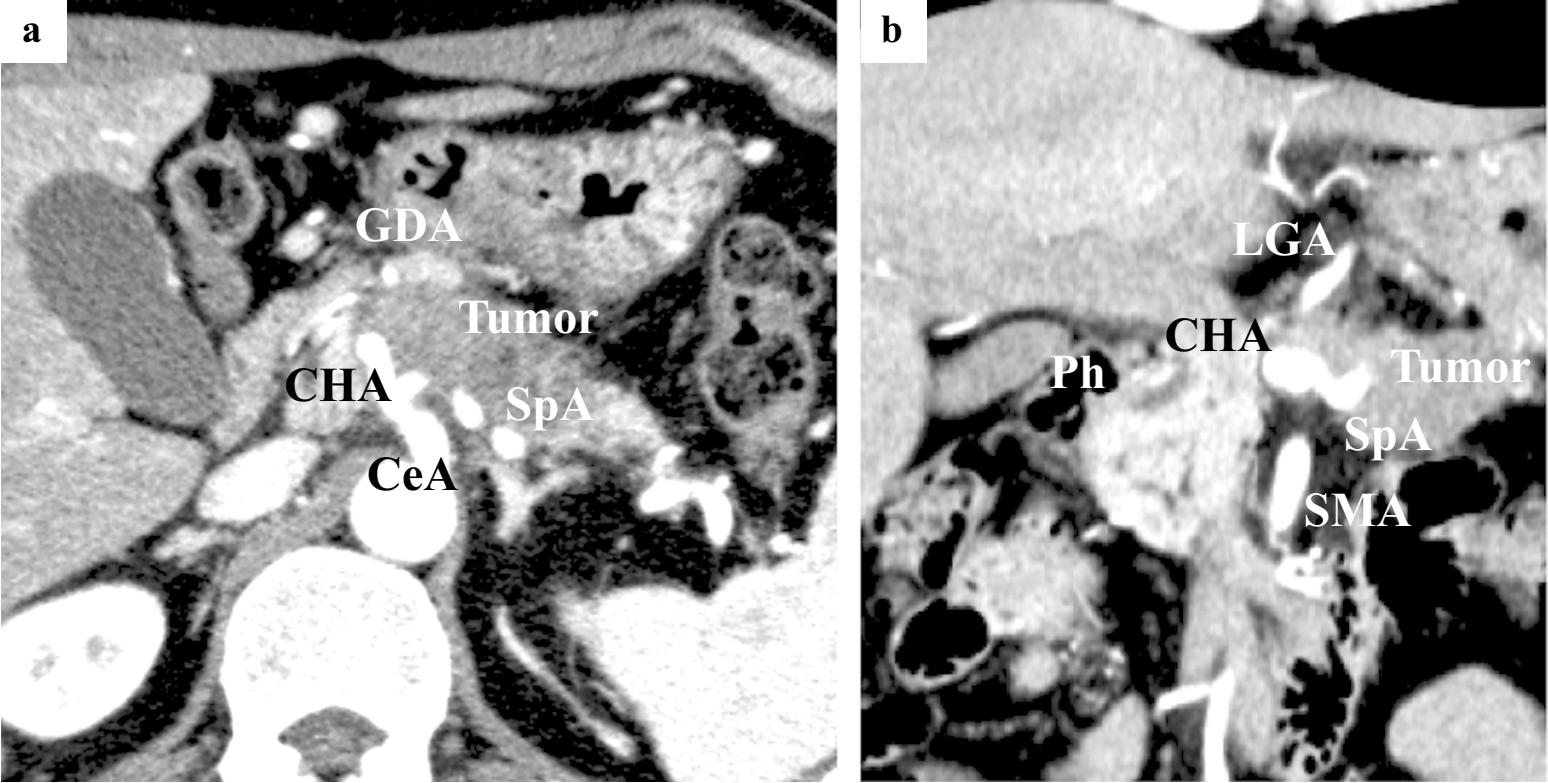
Abdominal CT imaging at the initial diagnosis. a 54-mm hypovascular tumor in the body to tail of the pancreas. b The main tumor in contact with the CeA, CHA, LGA, SpA and GDA
